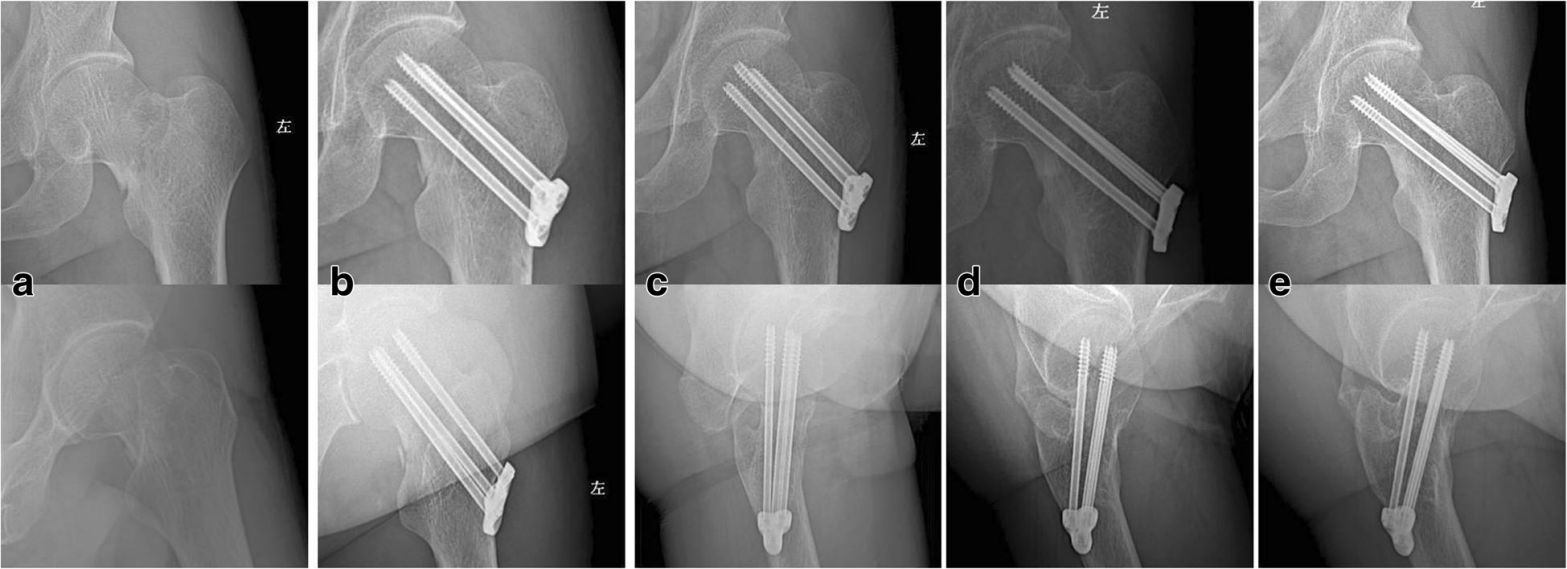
A 54-year-old man with a displaced left femoral neck fracture fixed with dynamic compression locking system. a Preoperative X-ray. b Postoperative X-ray review at 2 days after operation. c Postoperative X-ray review at 1 month after operation. d Postoperative X-ray review at 4.5 months after operation. e Postoperative X-ray review at 18.5 months after operation
